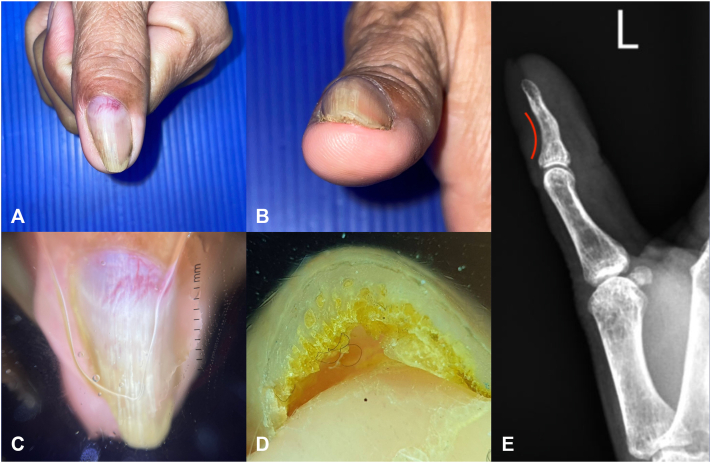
Clinical, dermoscopic, and radiographic images. A,B, The left thumbnail has distal onycholysis with an overcurvature on one side. C, Dermoscopy shows a structureless purplish area with prominent vasculature overlying the area of the lunula and longitudinal parallel white lines starting from the border of the lunula going toward the distal edge of the nail plate. D, Honeycomb-like cavitations are visualized on the free edge of the nail plate. E, X-ray views of the left hand showed scalloping (*red curve*) of the outer cortical margin of the dorsal distal phalanx of the thumb with a surrounding ill-defined soft tissue density with no internal calcification.
